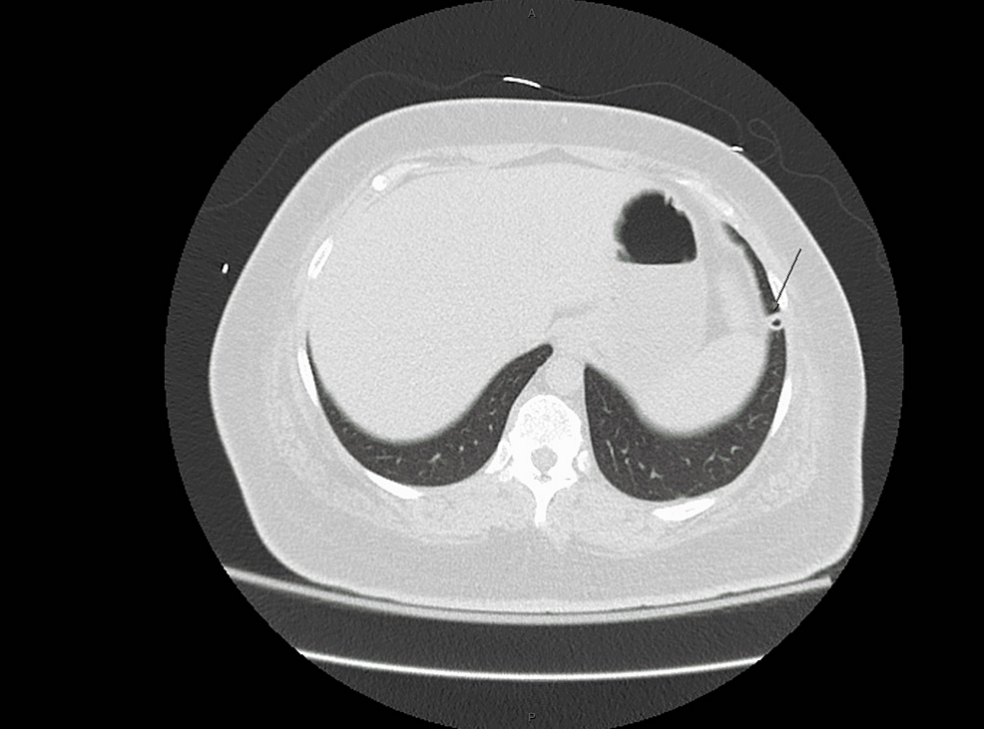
Computed tomography without contrast of the chest in transverse view. Black arrow indicates left pulmonary nodule.
